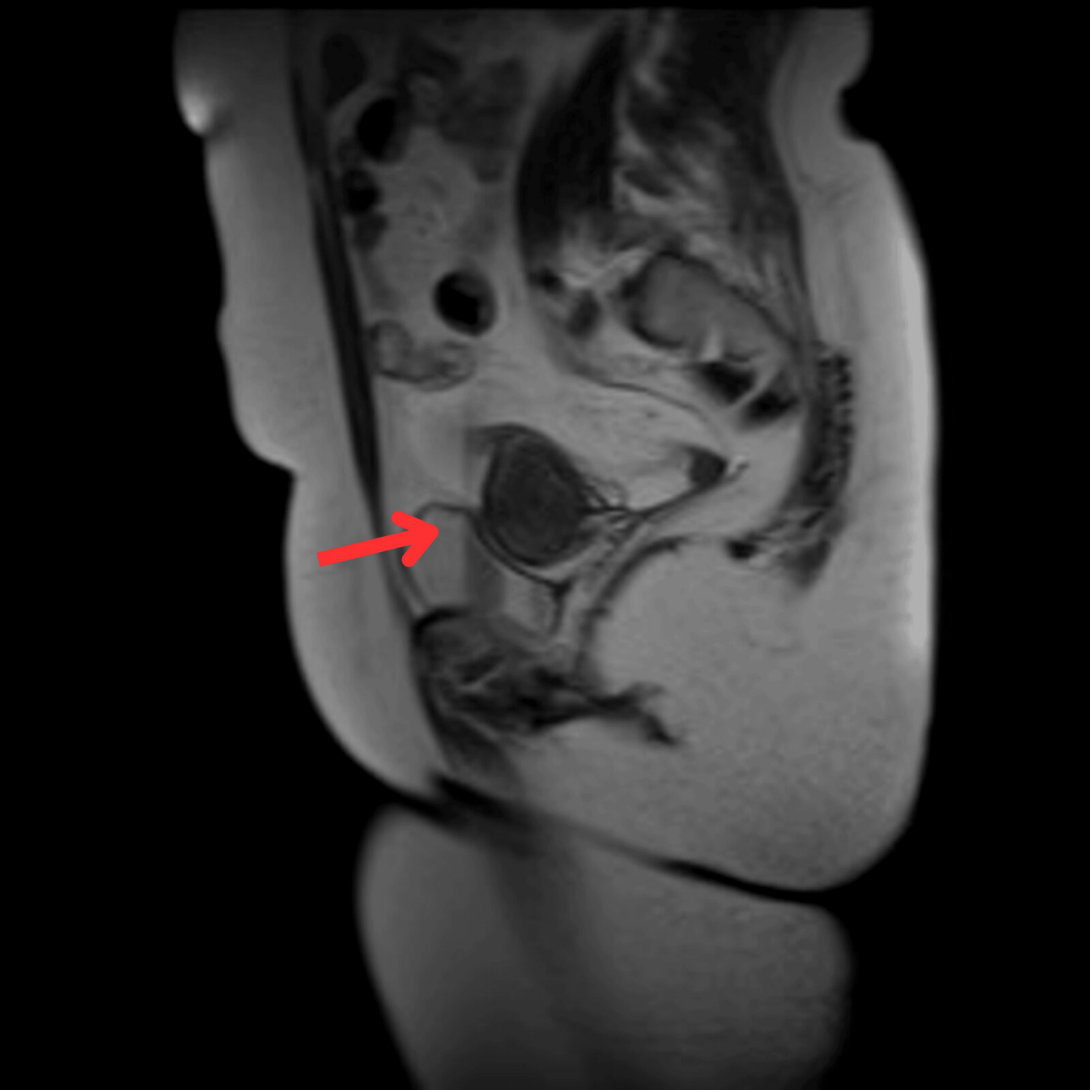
MRI showing right adnexal mass Arrow: adnexal mass
